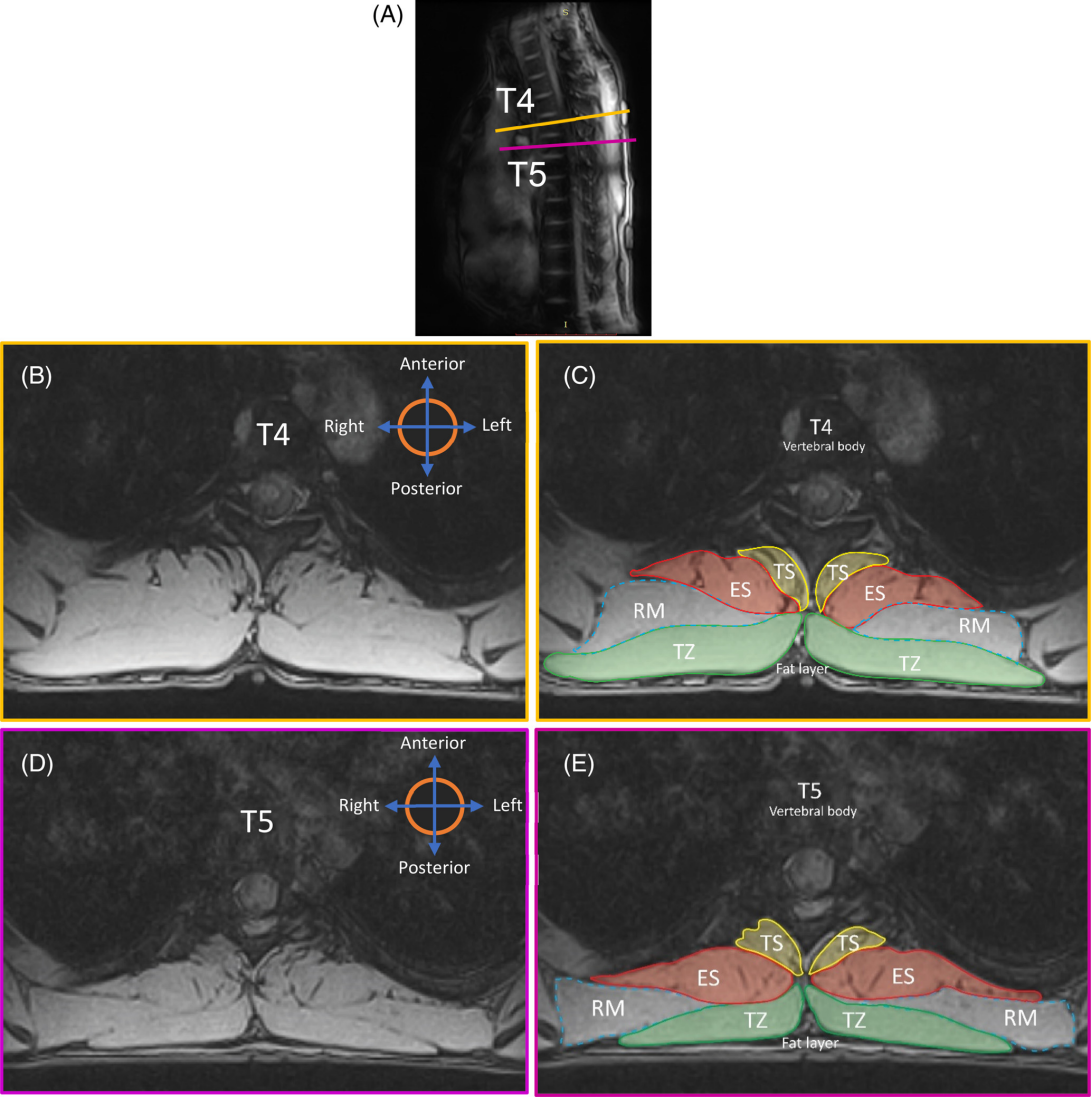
A, Sagittal MRI image showing the slice position and orientation in the thorax. B, Transverse MR image at mid T4 Level. C, Segmentation of the ROI at mid T4. D, Transverse MR image at mid T5 Level. E, Segmentation of the ROI at mid T5 level. ES, erector spinae; RM, rhomboid major; ROI, region of interest; TS, transversospinalis; TZ = trapezius
